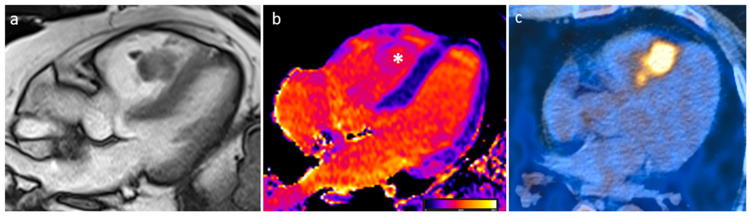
Right ventricular melanoma metastasis (*) with intermediate-high signal intensity on cine sequence (a), high native T1 mapping values (1600 ms) (b), and avid FDG uptake in PET-CT scan (c).
